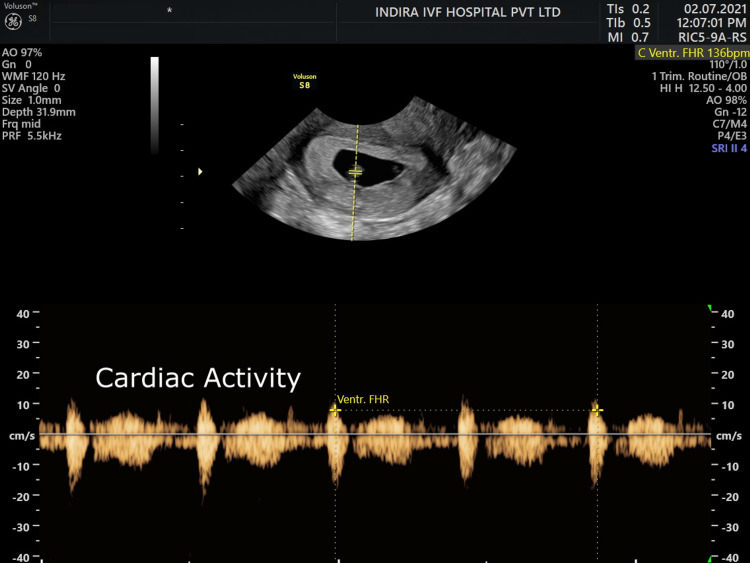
Ultrasound scan with fetal pole and cardiac activity.
